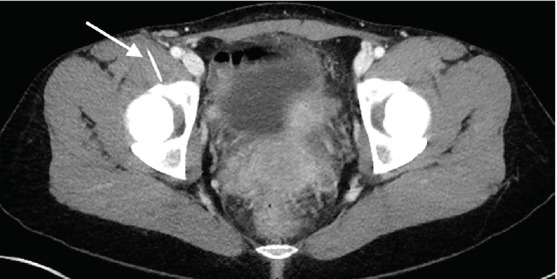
Computed tomography axial image demonstrating a radiopaque linear foreign body (arrow) passing through the iliopsoas muscle without gas or fluid along its path, resting adjacent to right anterior acetabular wall.
